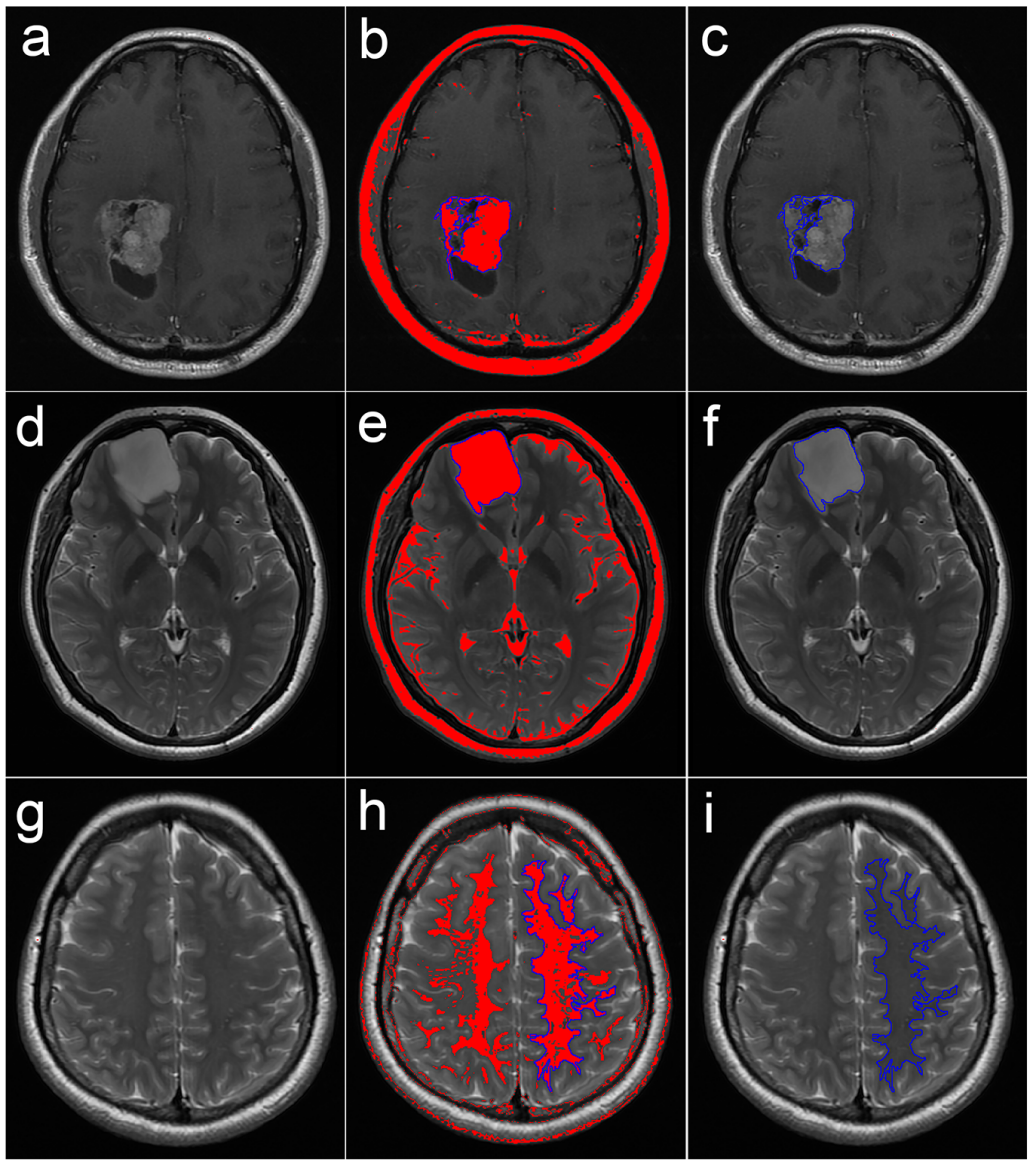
Semi-automated delineation of the ROIs over the solid region of the tumor and the NAWM a-c. Delineation over a solid enhancing tumor on transverse contrast-enhanced T1-FLAIR; d-f. delineation over a non-enhancing tumor on transverse T2-FSE; and g-i. delineation over a contralateral NAWM on transverse T2-FSE. When a proper threshold range of signal intensity was set, the corresponding pixels in the range were colored red, and then the wand tool was used to automatically delineate the connective pixels as the ROI (in blue). ROI: region of interest; NAWM: normal-appearing white matter; T1-FLAIR: T1 fluid-attenuated inversion recovery; T2-FSE: T2 fast spin echo.
